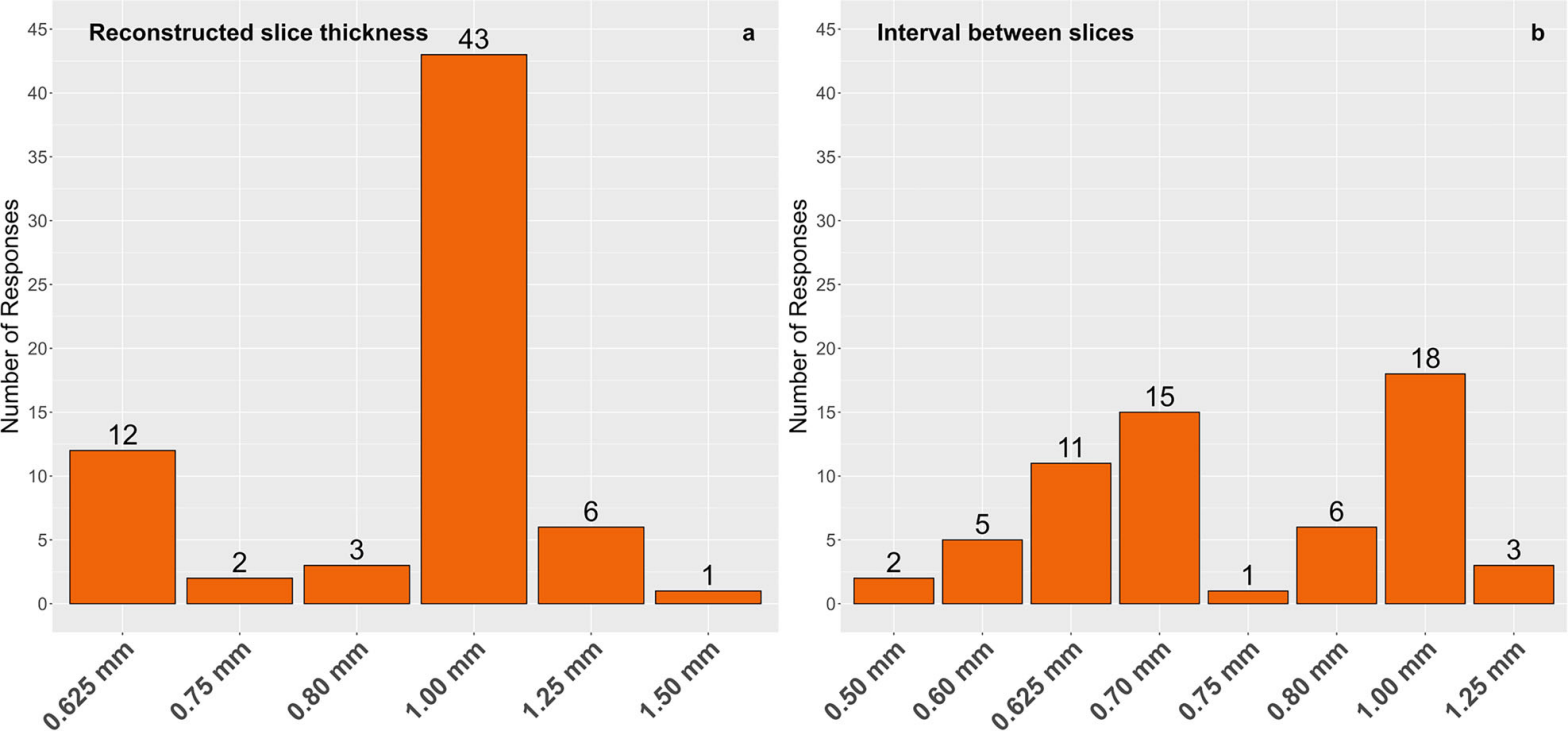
Slice thickness and interval in CT image acquisition protocols in lung cancer screening. Applied reconstructed slice thickness (a) and the interval between slices (b)
